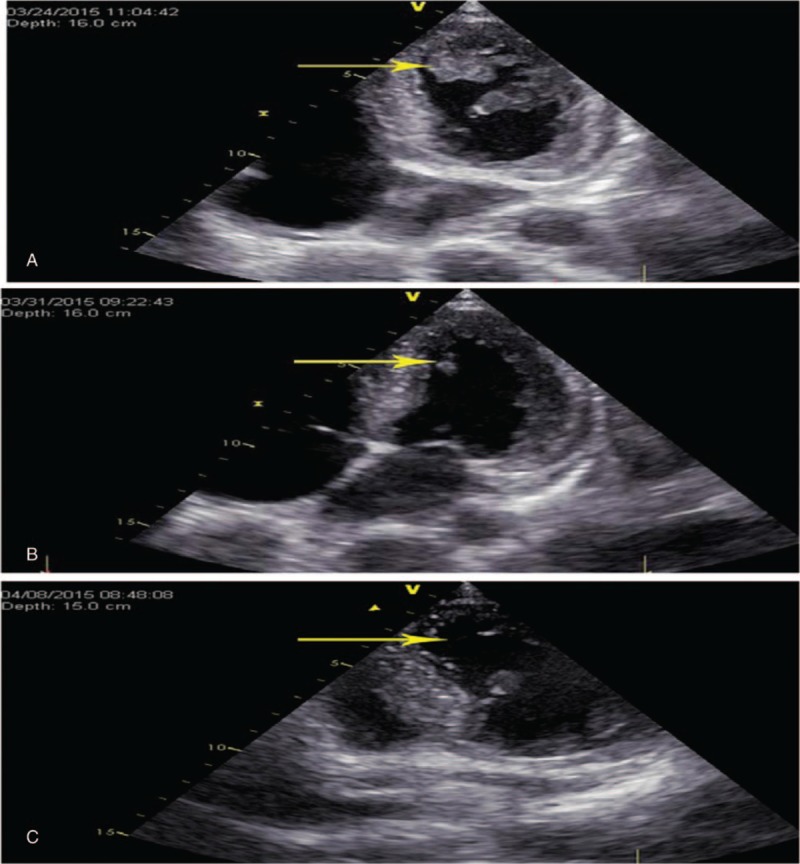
Changes in the apical thrombus visible on echocardiography.
